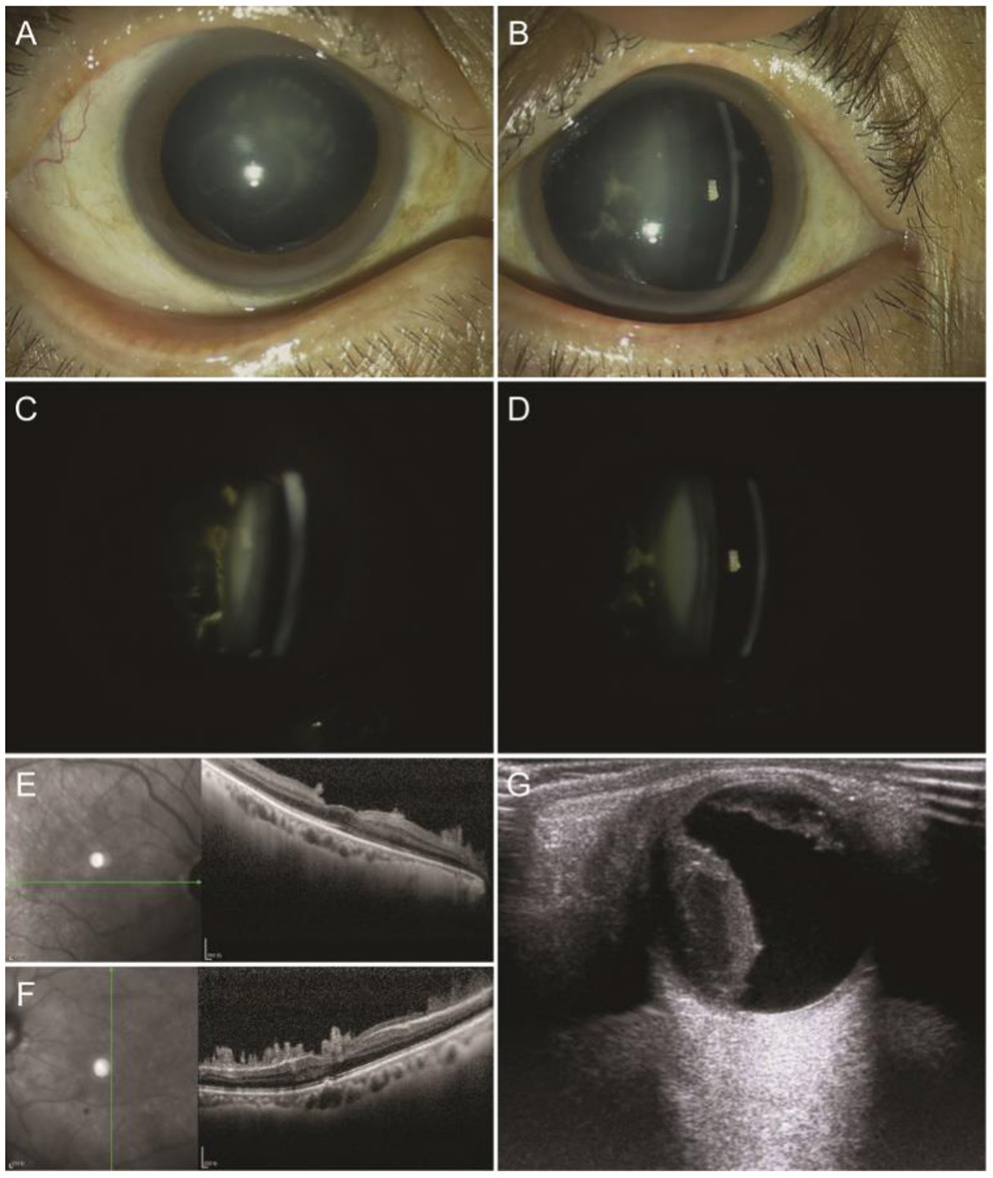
Images of the recurrent vitreous amyloidosis. Anterior segment images showed opaque nuclear with white heterogeneous opacity adhered to the posterior lens capsule. Optical coherence tomography (OCT) showed fern-like material adhering to the posterior pole retina surface. (A,C,E) Right eye; (B,D,F) left eye. (G) Color-Doppler ultrasound showed a dome shape temporal elevation of the retina with increased echo density in the right eye.
